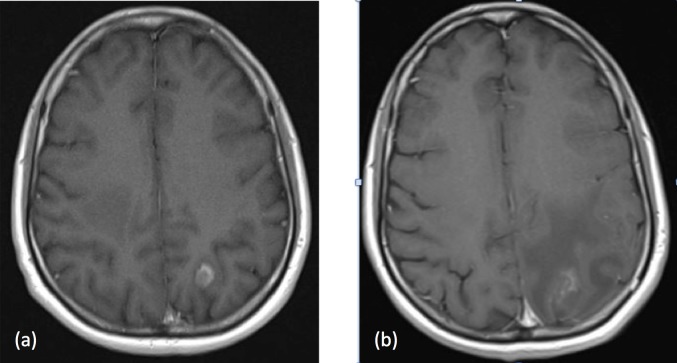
MRI head with a change in the left parietal lesion (a) Prior to SRS (May 2015): 10.2 mm x 12.4 mm; (b) Eight months following SRS (January 2016): 24.9 mm x 16.2 mm with peri-lesional edema MRI: magnetic resonance imaging; SRS: stereotactic radiosurgery
